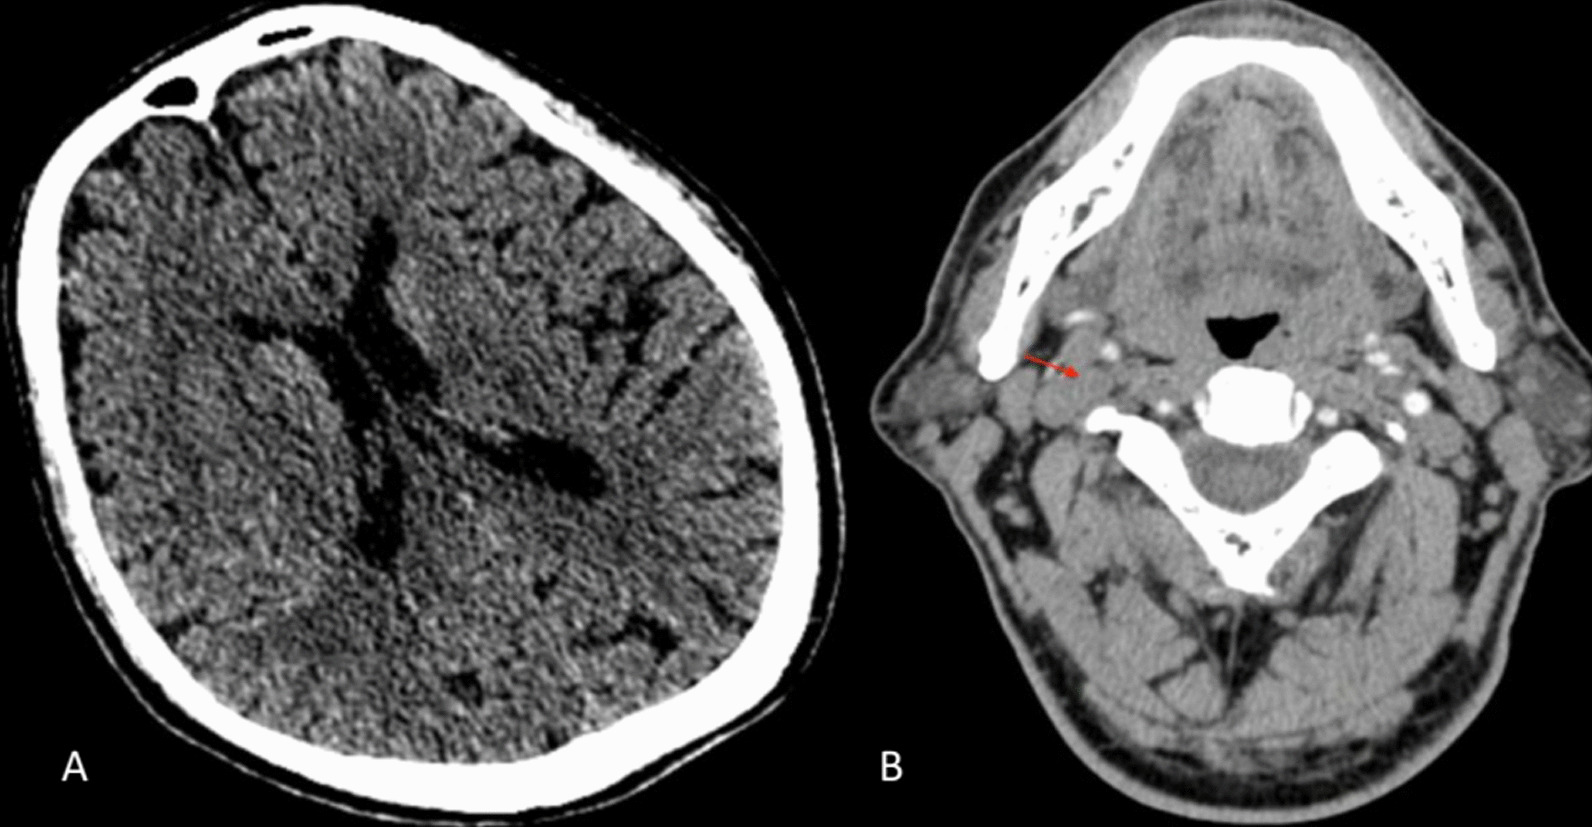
Noncontrast computed tomography (NCCT) showing early ischemic lesions on the right temporal lobe (A). CTA showed right ICA occlusion (red arrow) (B)
